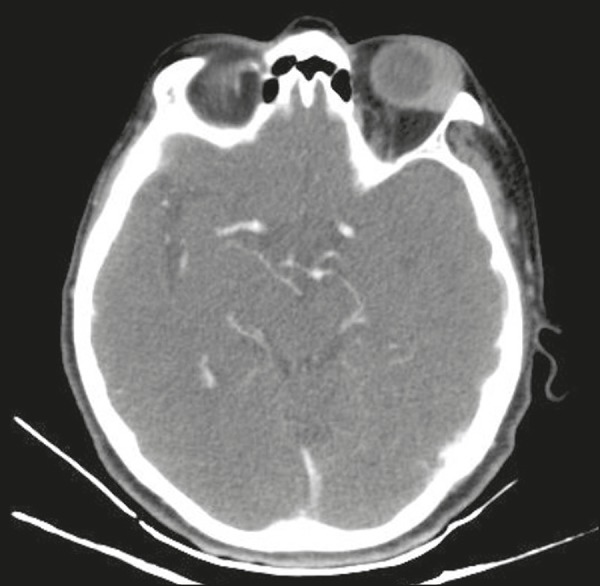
Contrast-enhanced axial CT scan showing lymphoma of the left ocular
